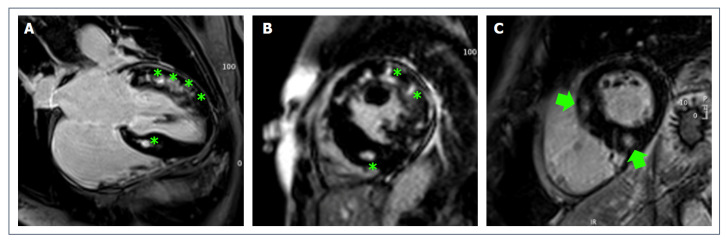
(A,B): post-contrast imaging (apical 4-chambers view and mid short-axis view) of a 32-year-old male athlete with a diagnosis of HCM with diffuse areas of patchy non-ischemic LGE (green asterisks). (C): short-axis view post-contrast imaging of a 41-year-old female ex-athlete with HCM showing areas of LGE in the basal anteroseptal segment and inferior RVIP (green arrows).
